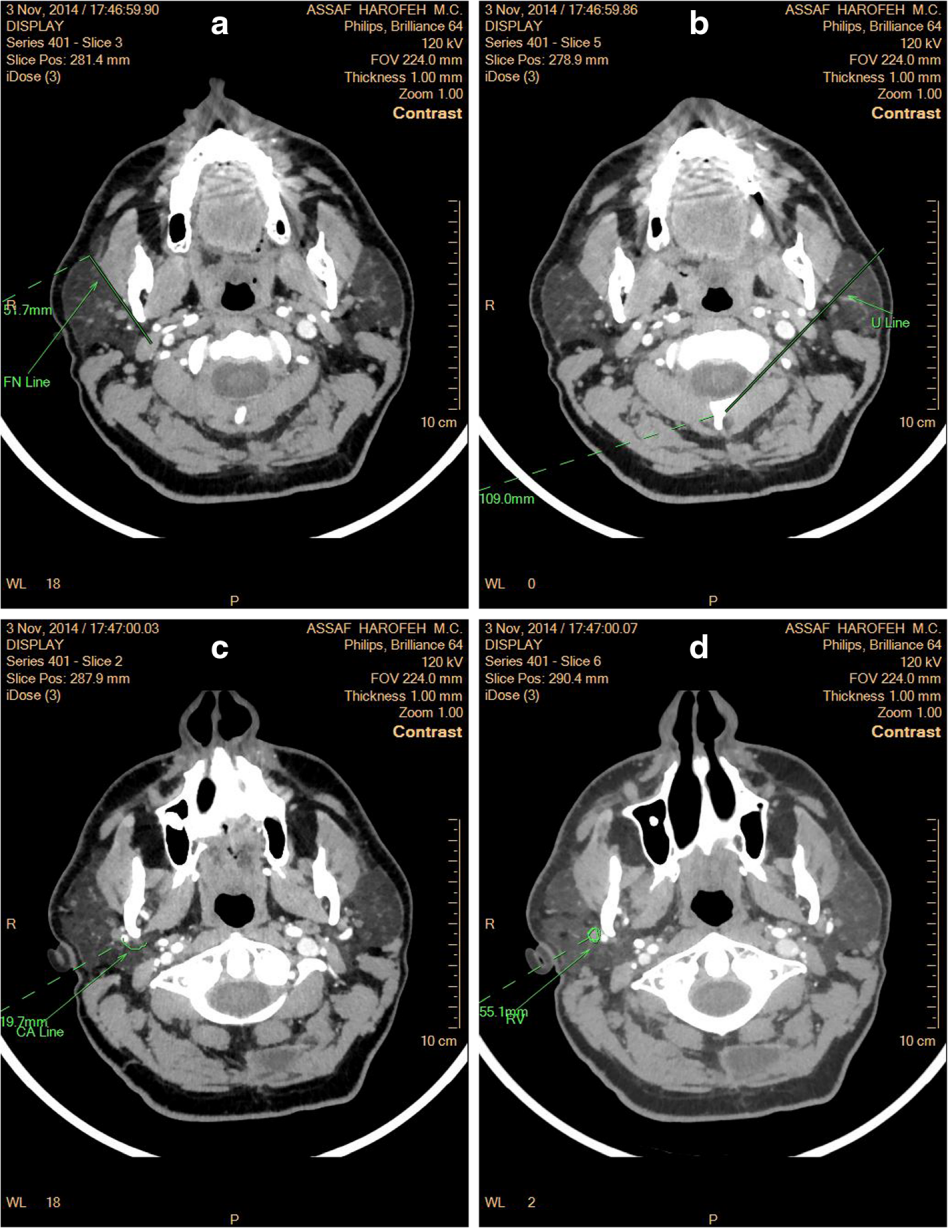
a The facial nerve line (FNL) is the line between the lateral surface of the posterior belly of *m. digastricus* and the lateral surface of the cortex of the ramus part of the mandible as seen at CT scan image. b The Utrecht line (UL) runs from the most dorsal point of the ipsilateral half of the first vertebra to the most dorsal point of the retromandibular vein as seen at CT scan image. c The Conn’s arc (CA) is a 8.5 mm radius semicircle with the center on the most distant point of the posterior edge of the ramus as seen at CT scan image. d The retromandibular vein line (RV) as seen at CT scan image
